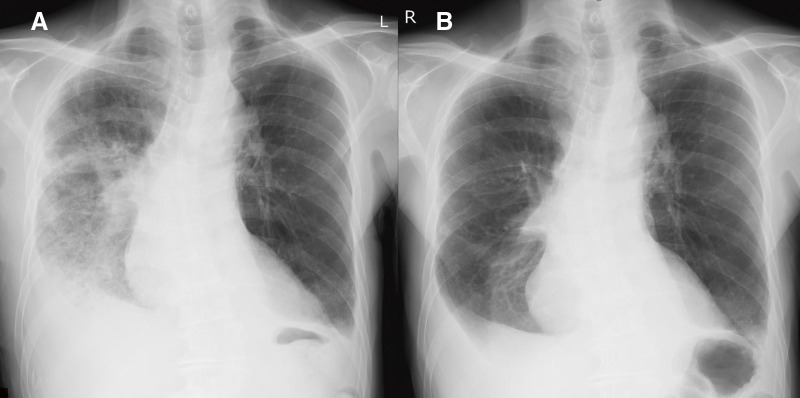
Postoperative radiograph. (A) Chest radiography taken on POD 6, before pulse steroid therapy, showing the infiltrate extending into the right residual lung. (B) Chest radiography taken on POD 77 showing recovery of the right lung from pneumonia. POD: postoperative day
